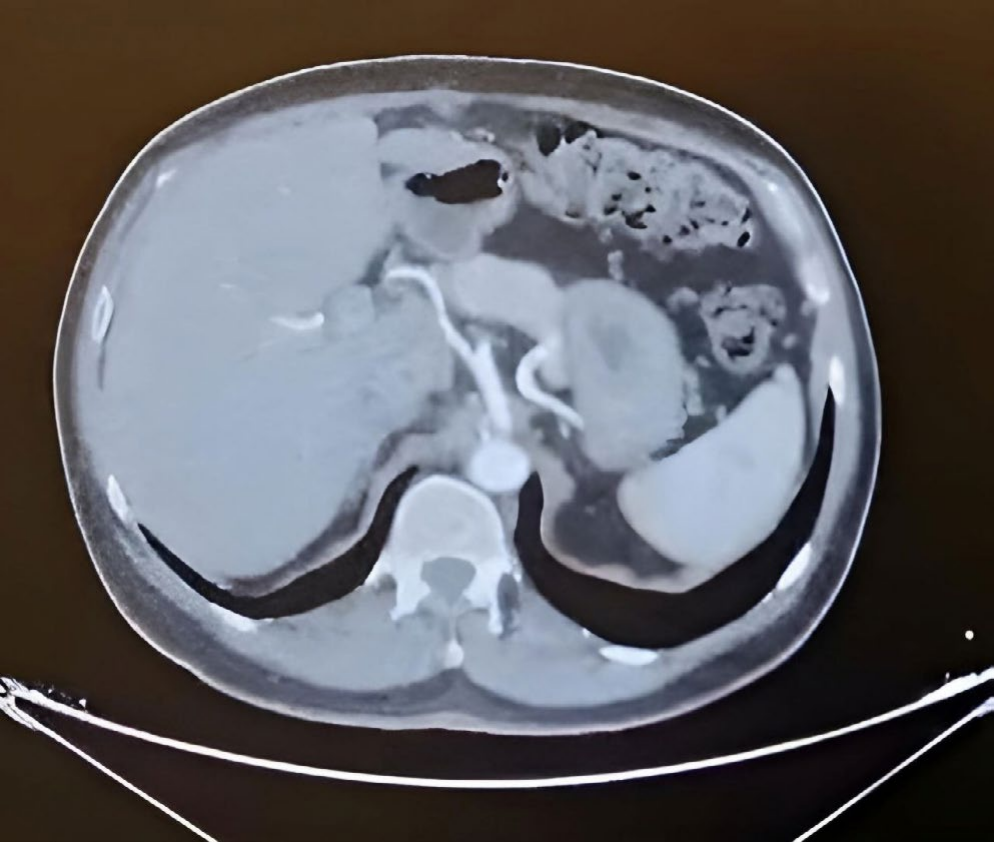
Axial contrast‐enhanced CT angiography (CTA) showing bifurcated endovascular stent graft after repair of infrarenal abdominal aortic aneurysm.
